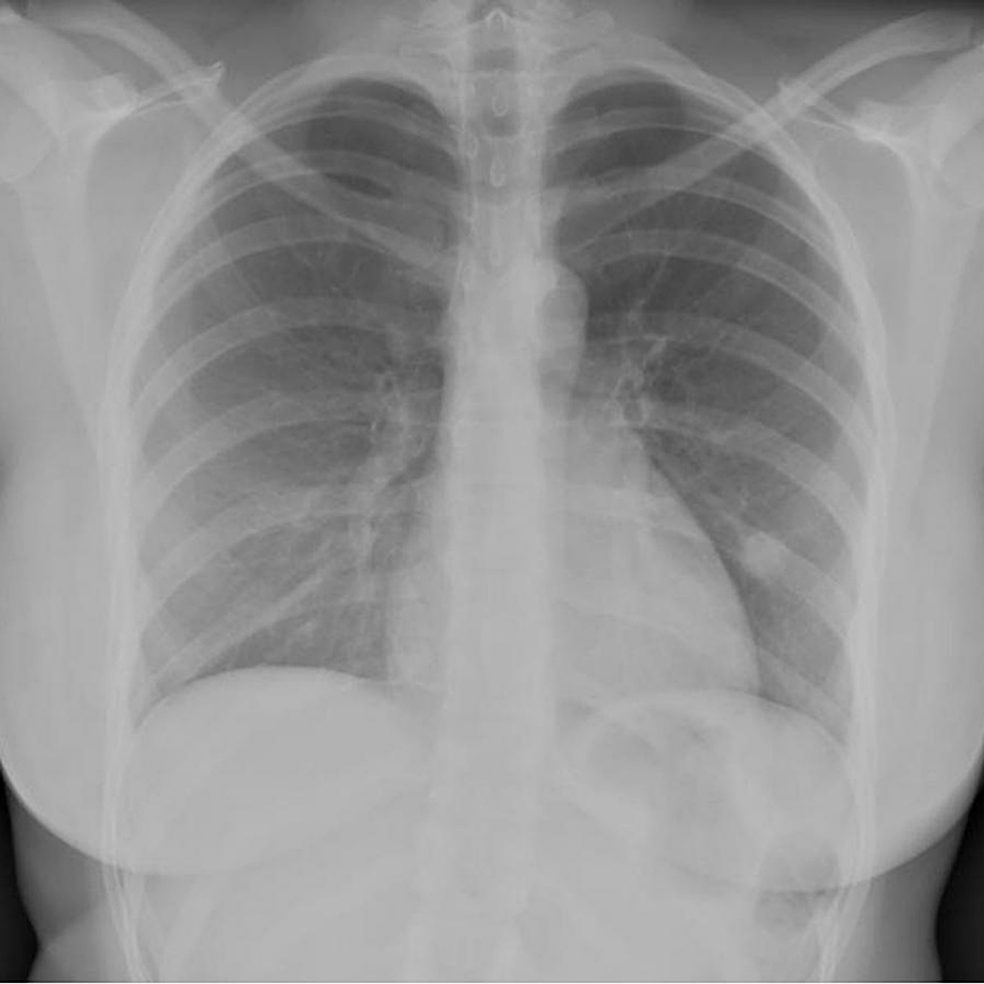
Chest radiograph of the patient showing a pulmonary nodule. The smooth, non-cavitating, non-calcified features of the nodule can be appreciated.
